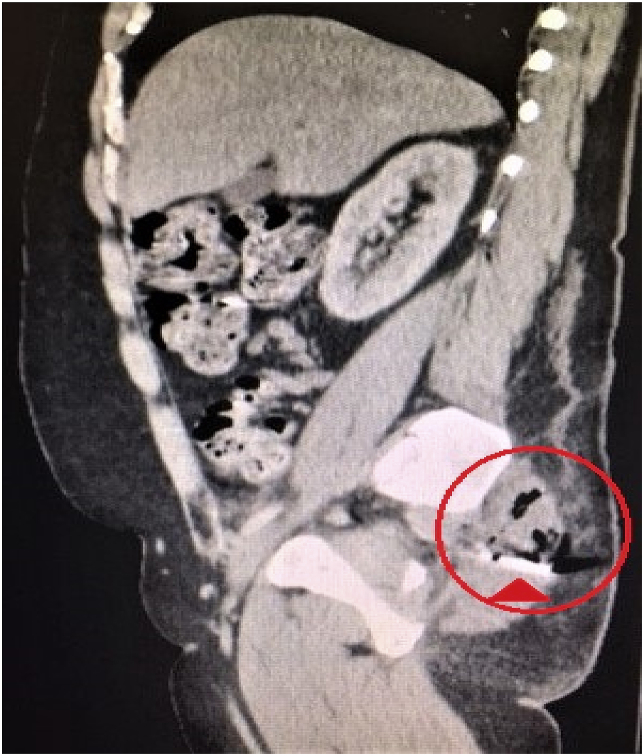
CT sagittal view showing the foreign body screw driver piercing through pelvic muscles with subcutaneous emphysema, circled in red with the arrow head showing the metallic end of the screwdriver.
